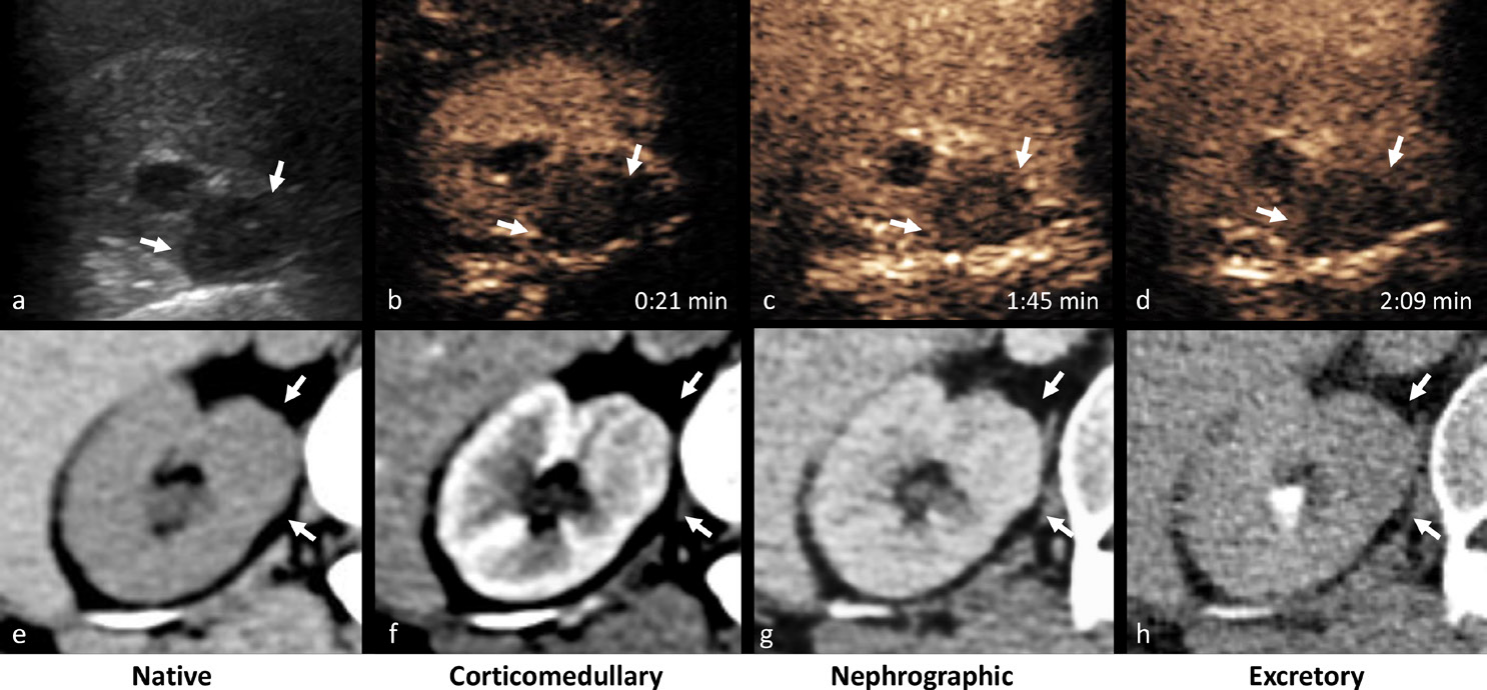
(a) Greyscale ultrasonography shows a hypoechoic cortical lesion in the medial aspect of the right kidney (arrows). CEUS obtained during the cortical (b) and parenchymal (c, d) phase reveals slow enhancement of the lesion which remains hypoenhancing to surrounding normal renal parenchyma. The lesion has the same attenuation as the adjacent renal parenchyma on unenhanced CT (e) and displays progressive enhancement on corticomedullary (f) and nephrographic (g) phase. CEUS, contrast-enhanced ultrasound.
